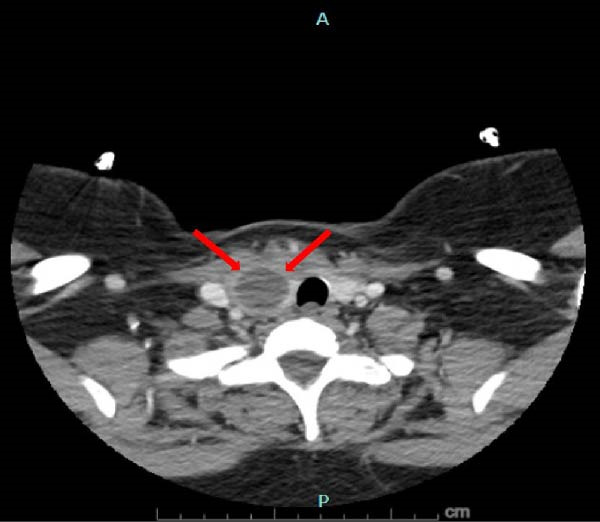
CT neck with red arrows demonstrating thyroid abscess formation.
